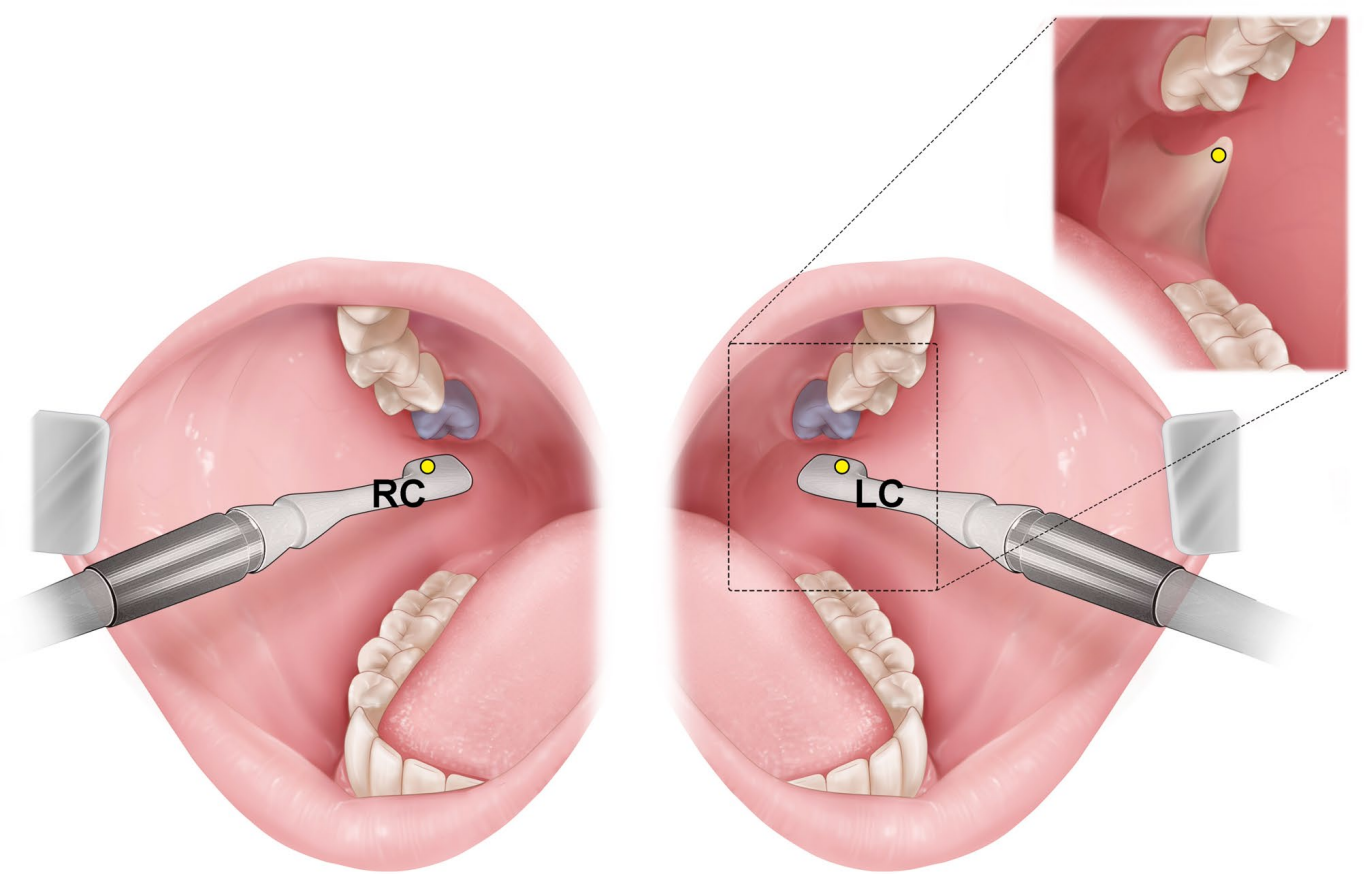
Reference point for the ultrasonography (US) scanning: LC, left cheek mucosa at the second molar (purple) on the occlusal plane; RC, right cheek mucosa at the second molar (purple) on the occlusal plane.
